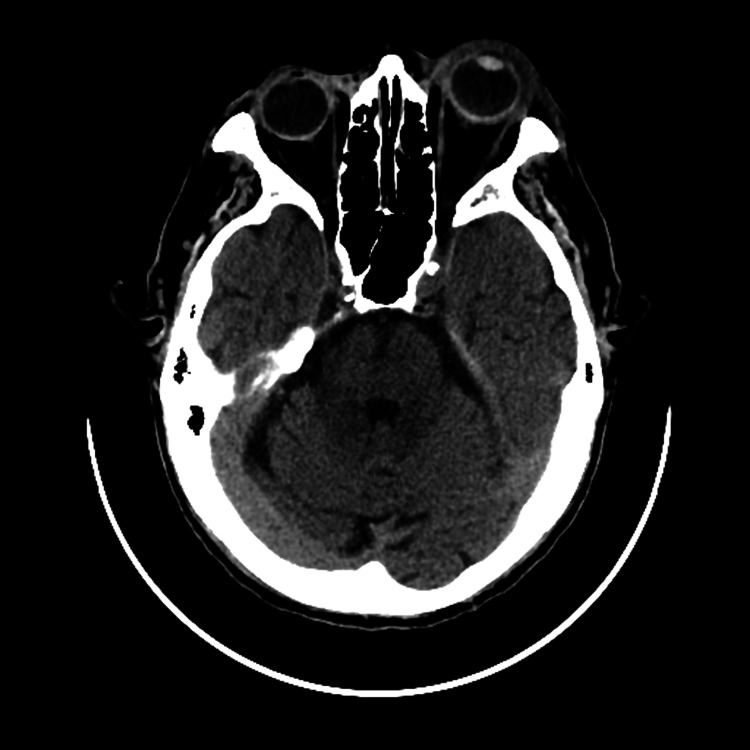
Head and orbital CT scan revealed, on the left side, orbital cellulitis, including a spontaneous hyperdense area interior to the orbital septum with contrast enhancement, retrobulbar fat densification, proptosis with optic nerve stretching, and chorioretinal thickening.
